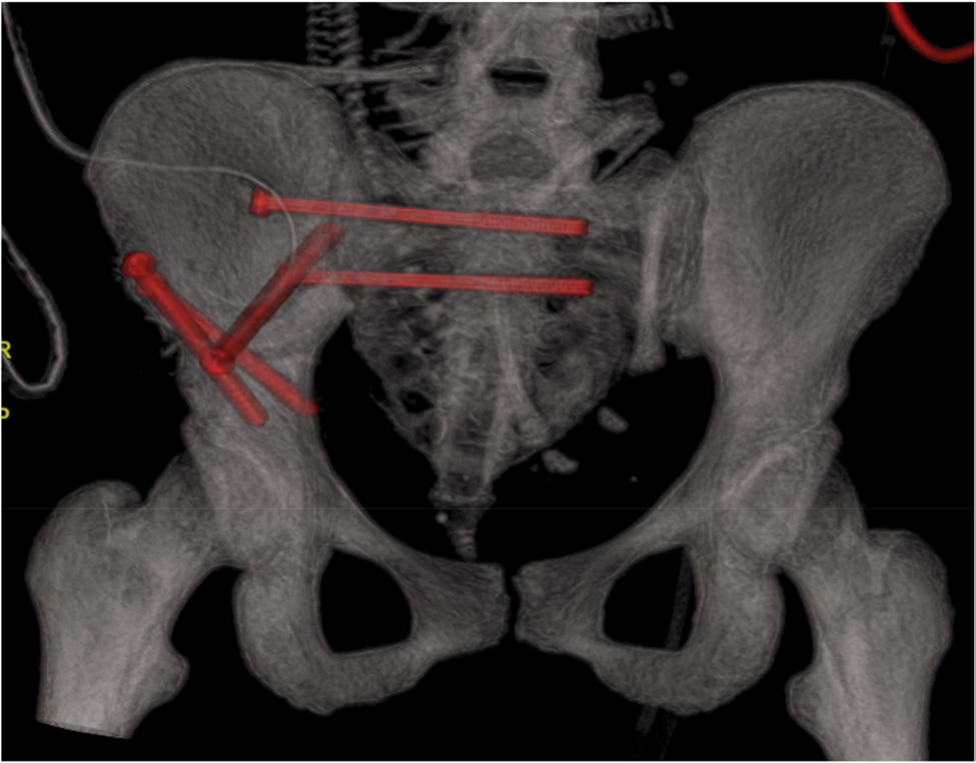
CT 3D reconstruction image of the postoperative pelvic state, 5 osteosynthetic screws in situ (labelled with red)
